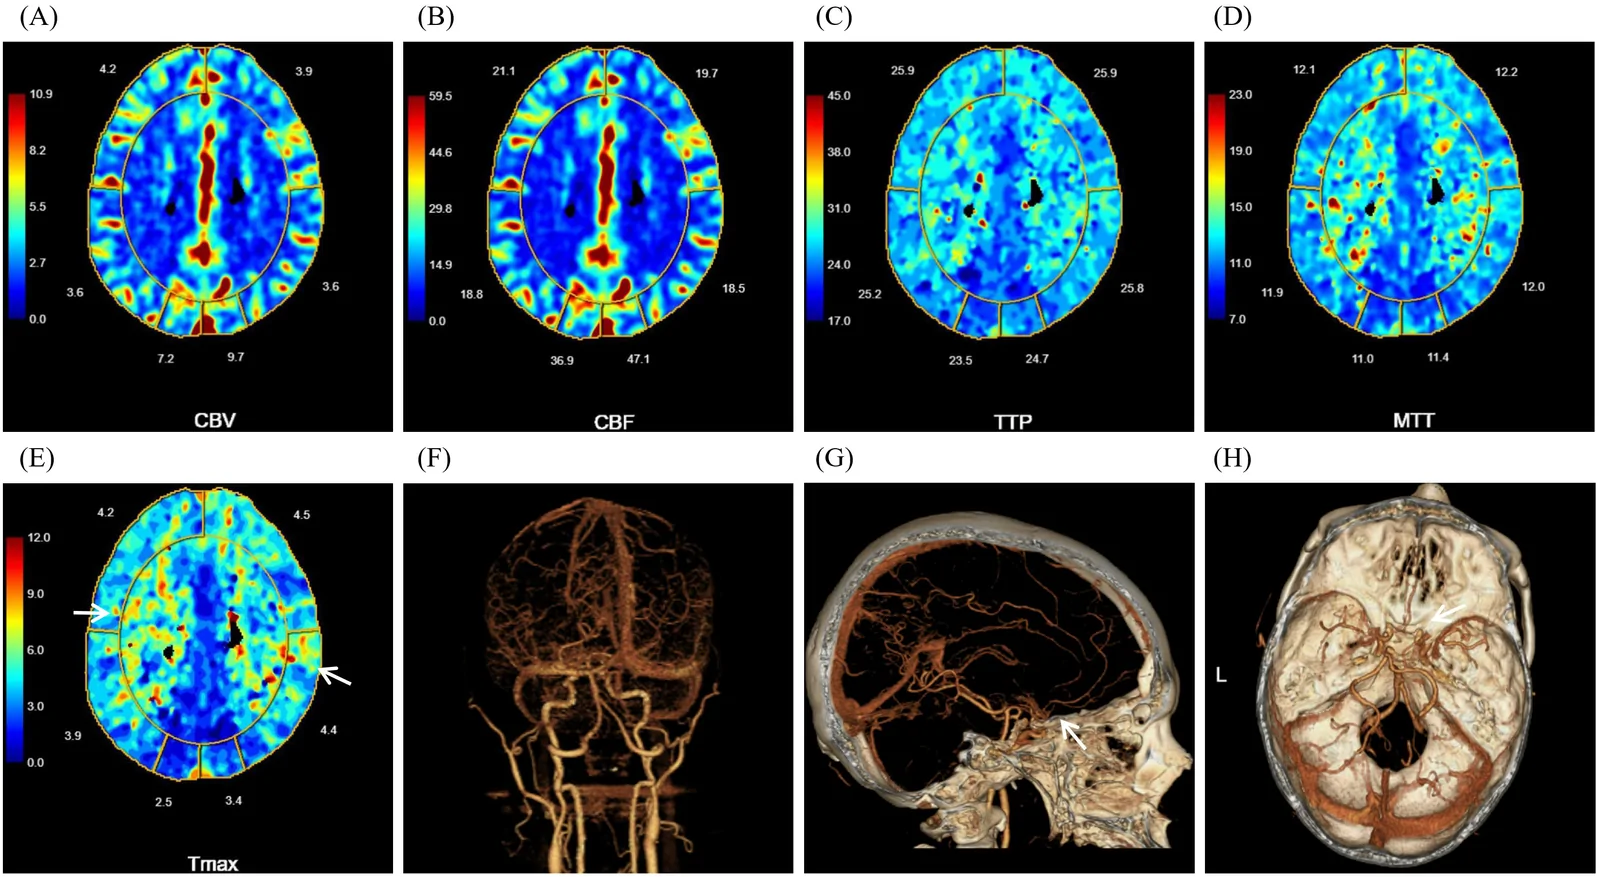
CT perfusion (CTP) and CT Angiography (CTA) images demonstrating characteristic imaging findings of MMD. (A–E) CBV shows compensatory elevation in the deep gray matter; CBF shows cortical hypoperfusion; MTT, TTP, and Tmax are diffusely prolonged, with a focal ischemic penumbra (indicated by yellow, orange, and red areas). (F–H) CTA reveals occlusion of the A1 segments of the bilateral anterior cerebral arteries and the M1 segments of the bilateral middle cerebral arteries, with surrounding “puff of smoke” collateral vessels (indicated by white arrows). The disease was classified as Suzuki stage III. (CBV, cerebral blood volume; CBF, cerebral blood flow; MTT, mean transit time; TTP, time to peak;Tmax, time to maximum; MMD, moyamoya disease).
